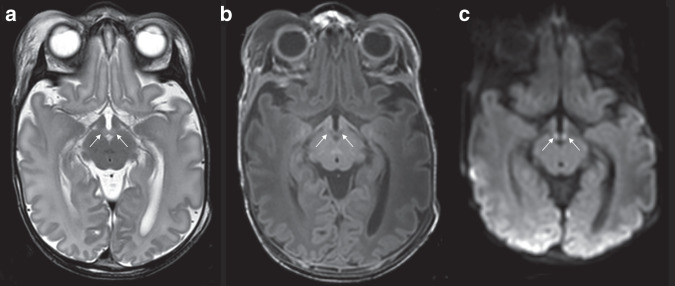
Infant with abnormal mammillary bodies. Abnormal mammillary body signal intensity and swelling on T2 (a) and low signal compared to normal-appearing white matter on T1 (b) and high signal on trace map of DWI (c) in a male infant, born 39 weeks GA, with perinatal asphyxia and treated with therapeutic hypothermia.
